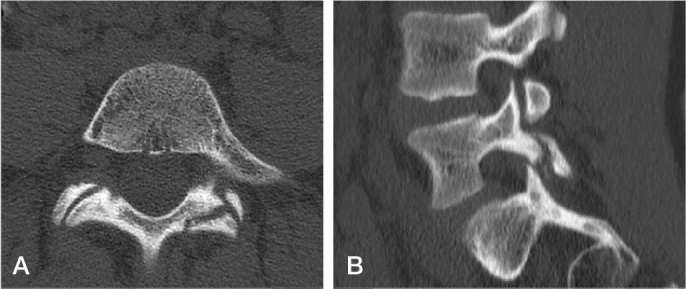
Progressive unilateral spondylolysis in the pars interarticularis of the L5. (A) axial CT, (B) sagittal reformatting.
